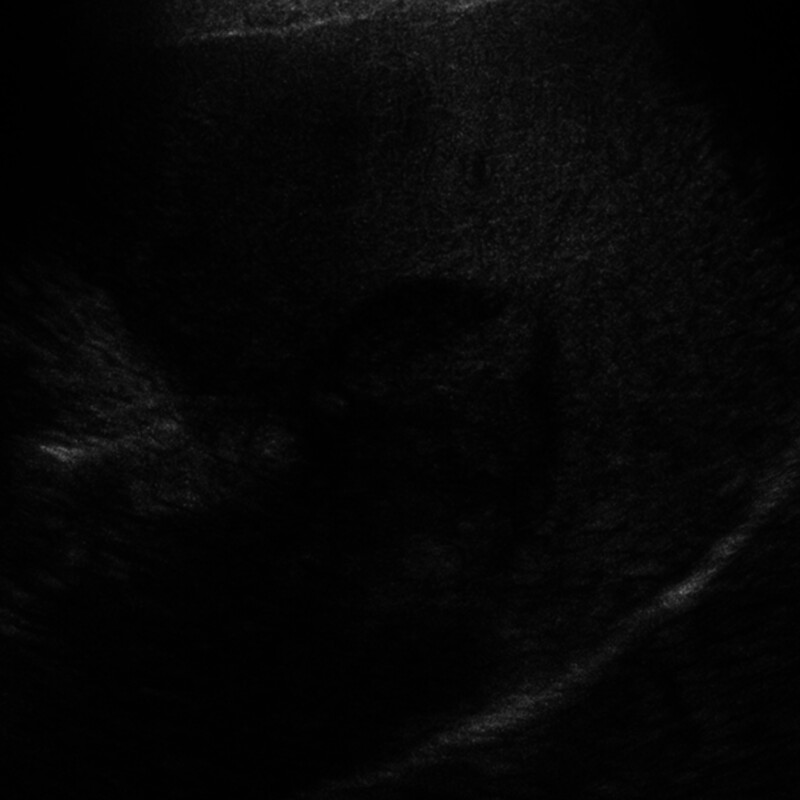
Follow-up abdominal ultrasound showing a stable 2 cm hepatic adenoma.
